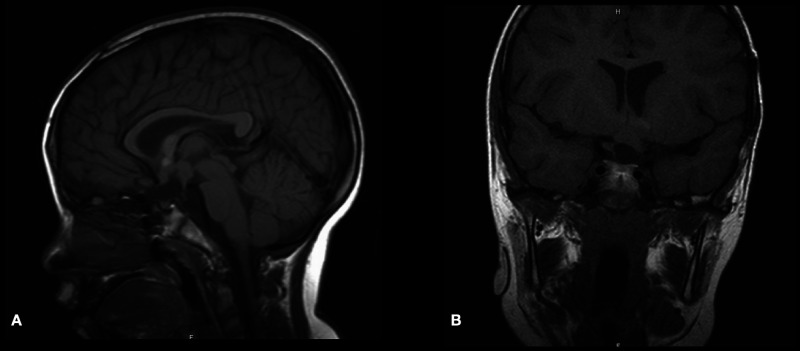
Postoperative sagittal and coronal T1-WI MRI scan demonstrating stable residual tumor at 5-year follow-up.
